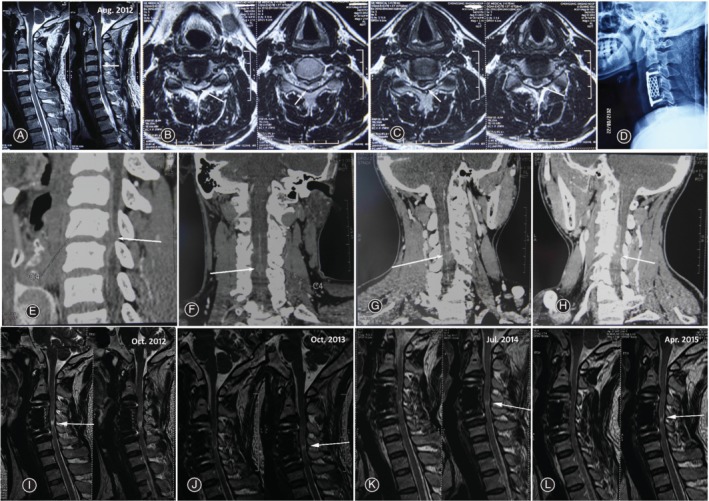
A 31‐year‐old man with ASAS and cervical spondylosis at C_3,4_ and C_4,5_. (A) Sagittal T2‐weighted MRI reveal that the cervical spinal cord was compressed at C_3,4_ and C_4,5_ (arrows). Spinal cord hyperintensity is showing from C_3_ to C_5_ (arrows). (B, C) Transverse T2‐weighted MRI showing spinal cord was compressed at C_3,4_ (B, arrows) and C_4,5_ (C, arrows). (D) Lateral radiograph view of anterior cervical corpectomy and fusion 3 days after surgery. (E, F, G, H) Sagittal view (E), coronal view (F), left oblique sagittal view (G), and right oblique sagittal view (H) of CT angiography showing anterior spinal artery occlusion at C_4,5_ (arrows). (I, J, K, L) The follow‐up MRI from 2 months (I), 14 months (J), 23 months (K) to 32 months (L) showing normal T2‐weighted imaging except for an area of myelomalacia cord at C_4,5_ (arrows).
